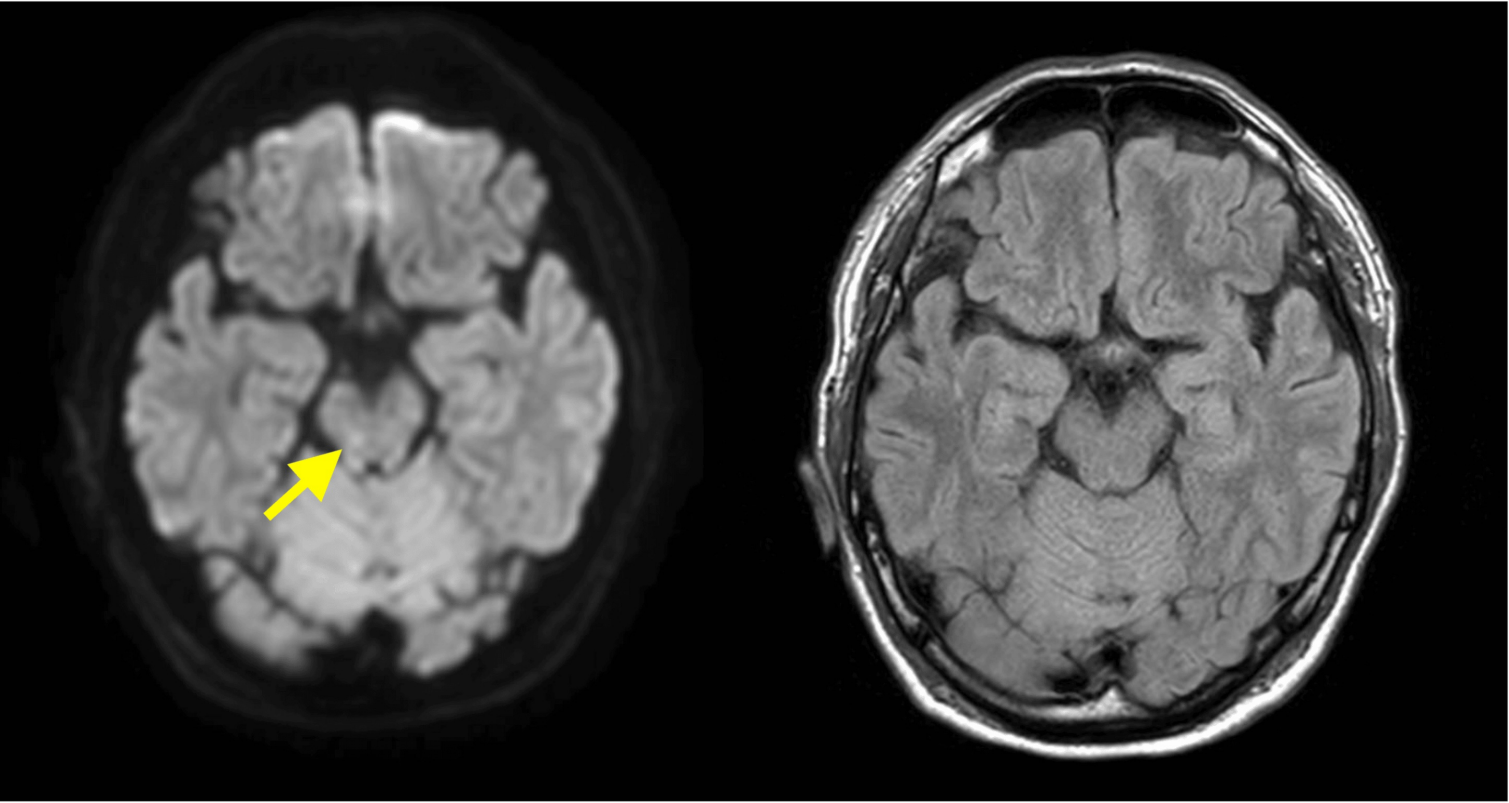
Diffusion-weighted imaging (DWI) on brain MRI (left) shows a midbrain infarction indicated by a yellow arrow. Fluid-attenuated inversion recovery (FLAIR) imaging (right) does not clearly demonstrate the infarction.
